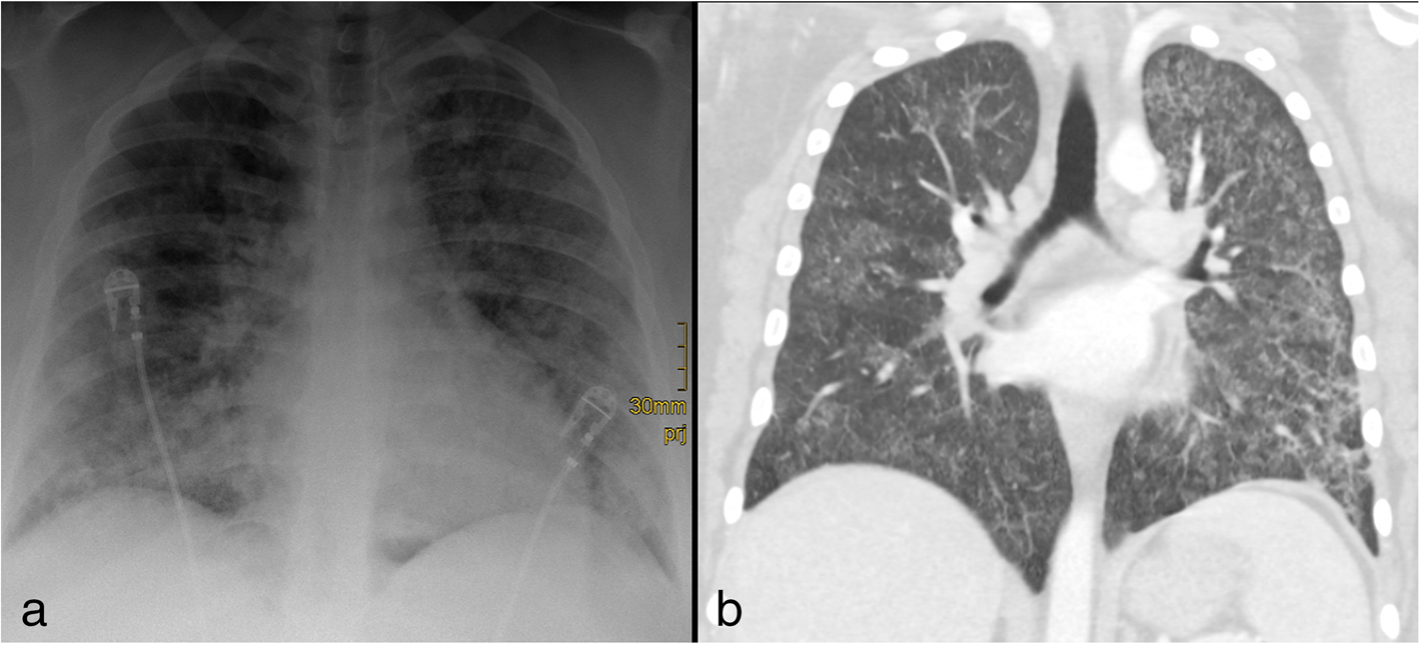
a Chest x-ray at admission showed diffuse ground glass opacifications with minimal peripheral consolidations. b Four days later a CT scan of the chest revealed diffuse bilateral ground-glass opacities with minimal peripheral consolidations
